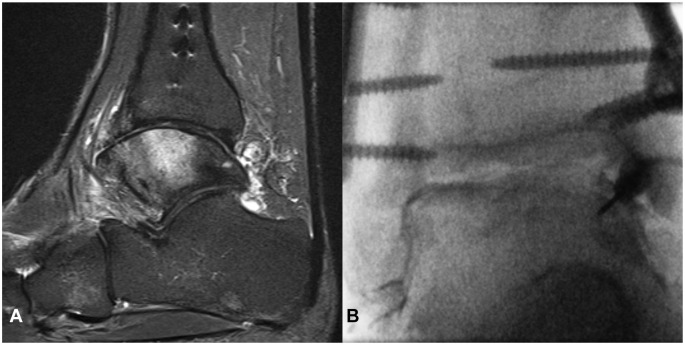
(A) Sagittal STIR magnetic resonance image before fluoroscopy-guided intraarticular injection of an ankle joint of a 29-year-old man with posttraumatic osteoarthritis shows severe bone marrow edema and severe cartilage damage in the ankle joint. (B) corresponding fluoroscopic image taken during injection. This patient responded very well to treatment, with a decline in VAS from 4 before to 0 after injection (100% decline). STIR, short tau inversion recovery; VAS, visual analog scale.
